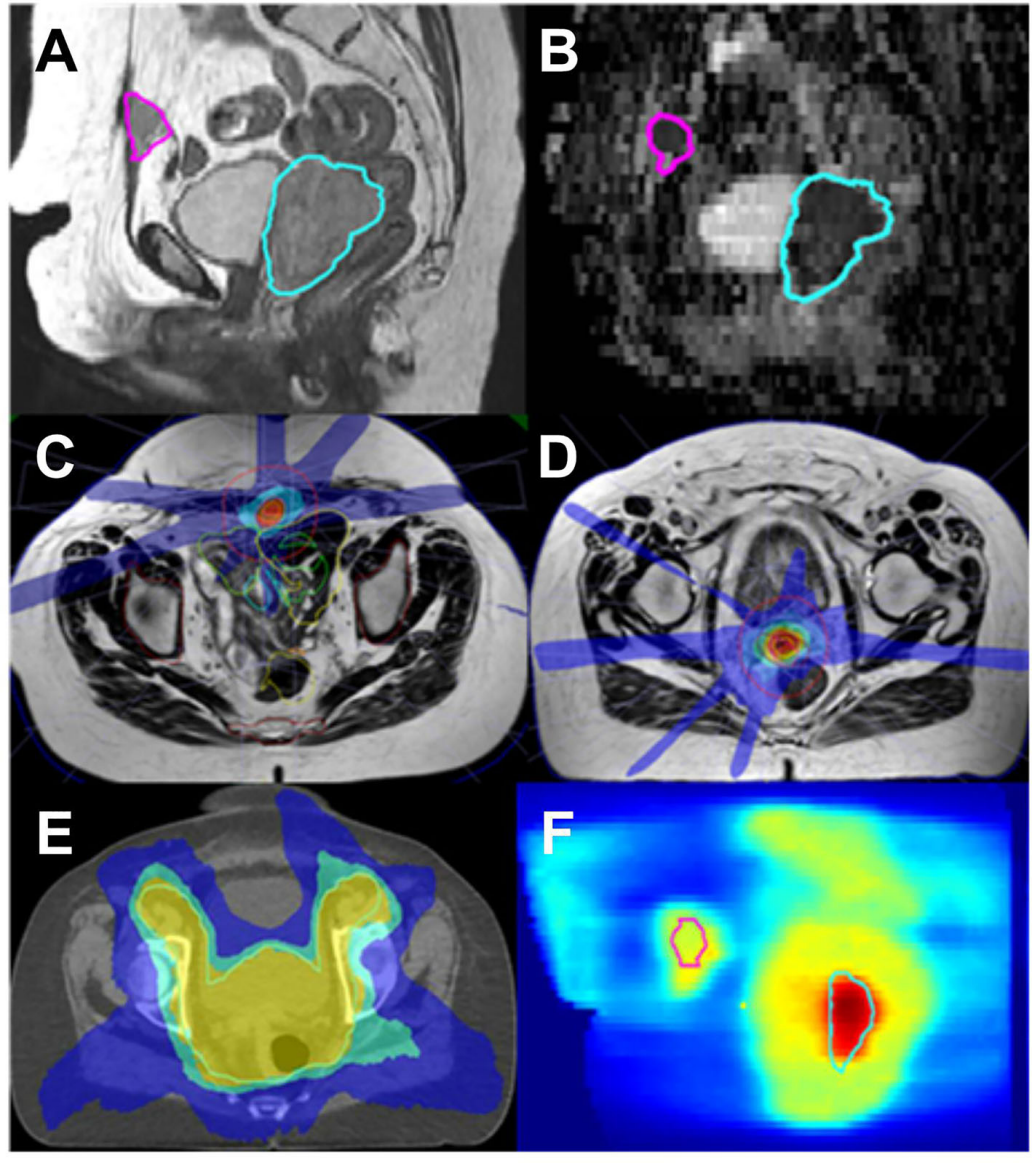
Endometrial cancer; MR Linac SBRT for concurrent vaginal vault recurrence and anterior abdominal wall metastasis. (A): T2 sagittal (B): ADC map derived from diffusion weighted acquired with MR Linac. Abdominal metastasis in pink and vaginal vault recurrence in blue. Target and OAR are clearly visualized on the MR images allowing for daily adaptation. (C, D): Typical daily MR Linac plans for both lesions (isodoses red 110%, orange 100%, blue 25%). (E): elective EBRT plan on planning CT (yellow 95%, green 82%, blue 52%) (F): overlay of elective and boost plans (range 0–70 Gy physical dose).
